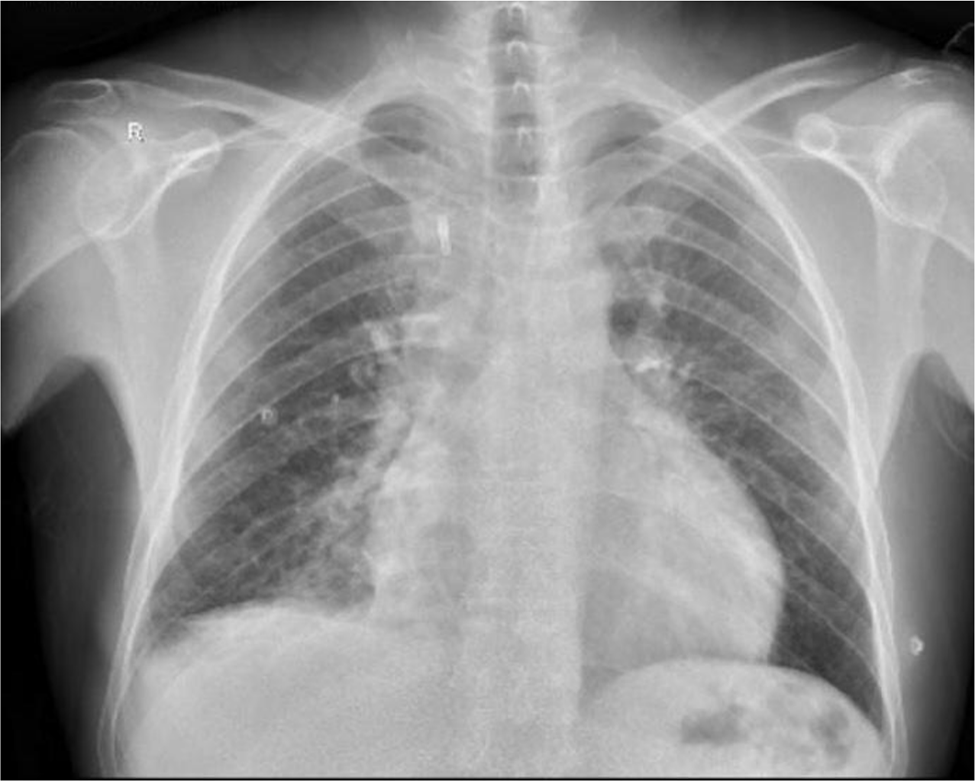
Chest X-ray (CXR) shows retrocardiac consolidation of the right lower lobe of lung, enlargement of the cardiac outline with water bottle sign suggesting pericardial effusion and obliteration of right costopherinc angle denoting moderate right-sided pleural effusion.
